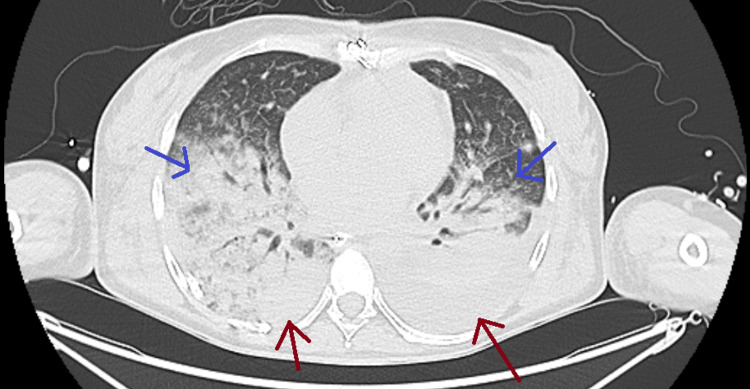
CT scan of the lungs Bilateral pleural effusions (red arrows) and basal atelectasis/consolidations (blue arrows). CT, computed tomography
